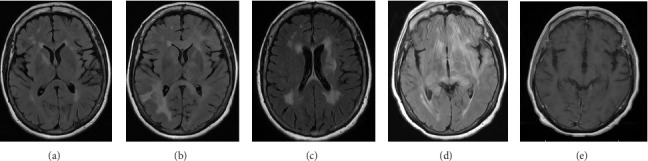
MRI images of different stages during disease course. (a): Imaging at symptoms onset. FLAIR sequence, white matter signals in the right temporal lobe, right anterior limb of the internal capsule/basal ganglia, and the corona radiata concerning for demyelinating lesions or acute disseminated encephalomyelitis. (b): Interim imaging after receiving methylprednisolone. FLAIR sequence, increased size of the right temporal lobe lesion, and multiple new areas of contrast enhancement in the periventricular area. (c): Interim imaging after receiving rituximab. FLAIR sequence, decreased size of the right peritrigonal lesion, an increased size of the lesion in left corona radiata extending into corpus callosum, basal ganglia, and subinsular region along with patchy enhancement and new enhancing lesions in the right thalamus and parietal cortex. (d): Imaging at admission. Postcontrast T1-weighted, worsening of known supratentorial lesions, and extension of lesions in infratentorial areas with new enhanced lesions in bilateral pericallosal/cingulate gyrus region, left corona radiata, left basal ganglia, and right lateral parietal lobe. (e): Imaging after hematopoietic cell transplant. Postcontrast T1-weighted, resolution of previously seen enhancing lesions.
